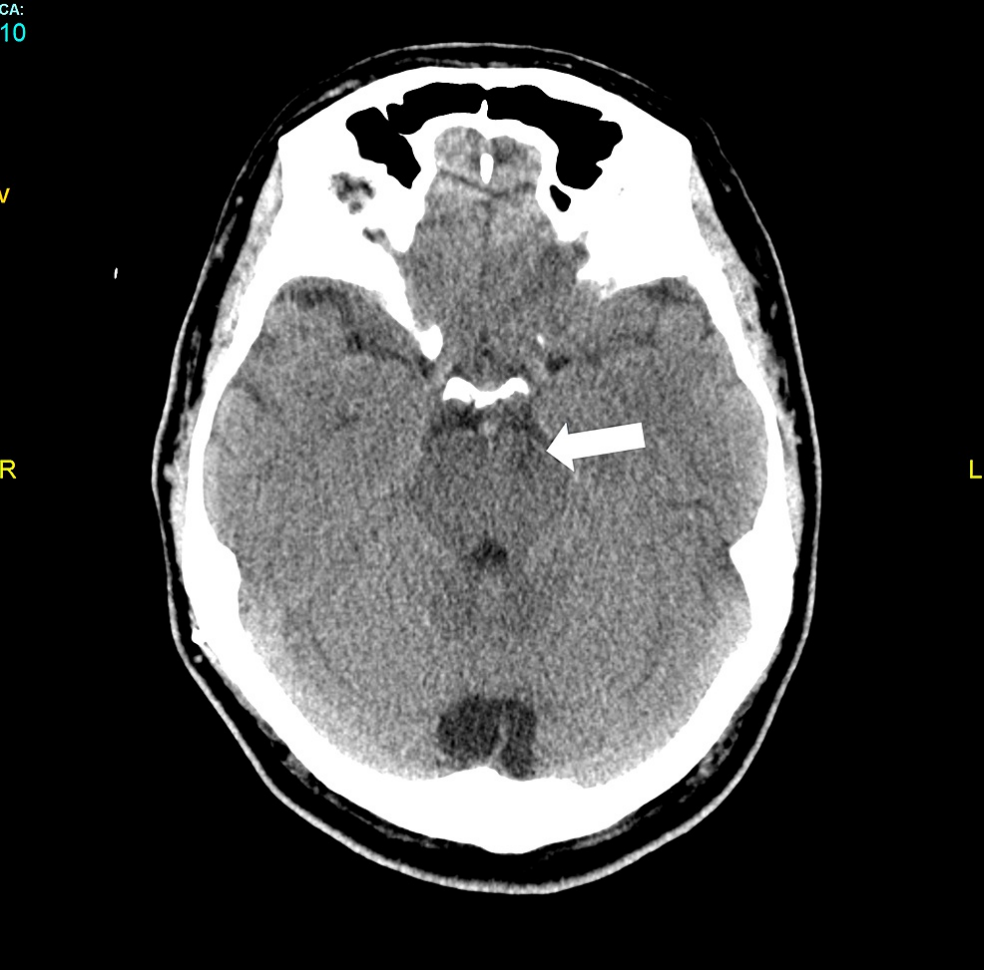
CT without intravenous contrast (axial plane) A small area of encephalomalacia is detected on the left cerebral peduncle (white arrow).
